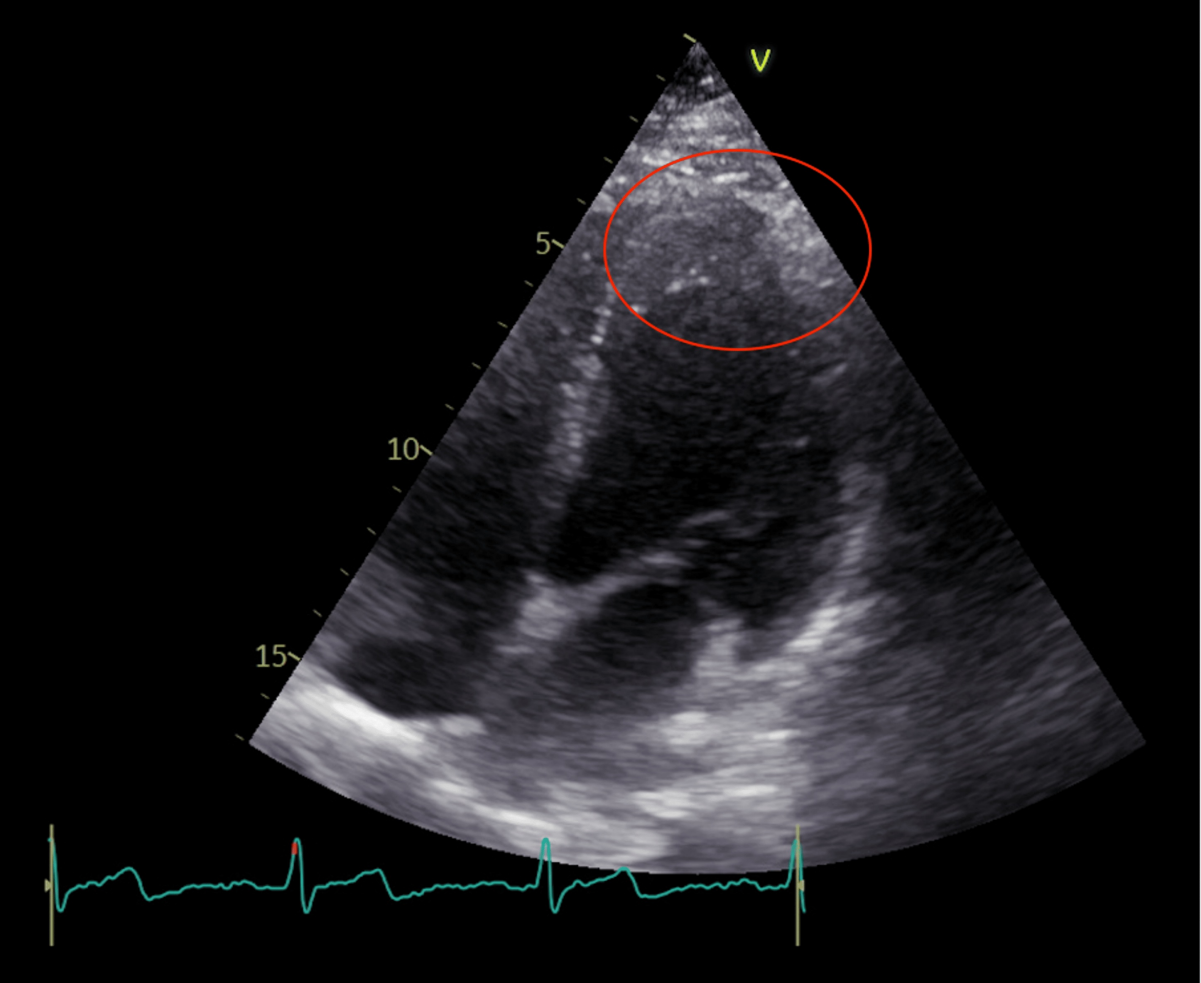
The apical four-chamber view showing increased thickness (12 mm) of the apex of the left ventricle
